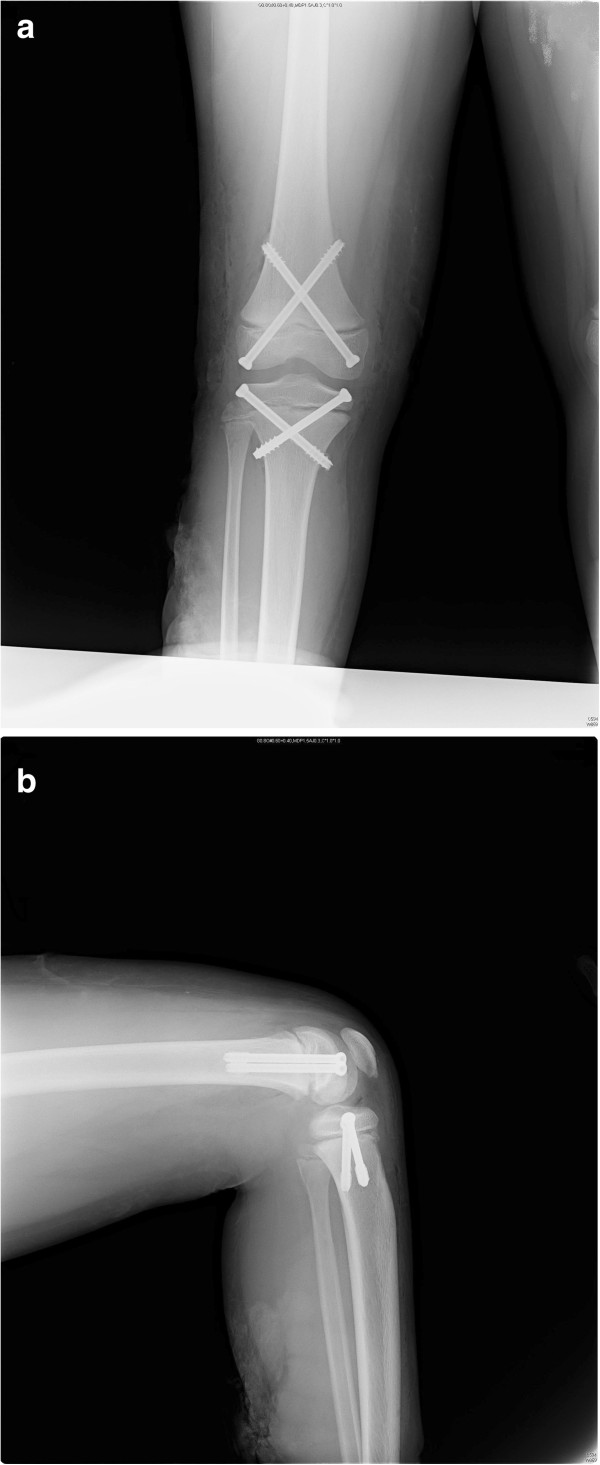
Radiological images of the patient at three weeks after the operation of his right knee demonstrating anatomic reduction. (a) Anteroposterior and (b) lateral radiographs of the knee at three weeks after fixation.
